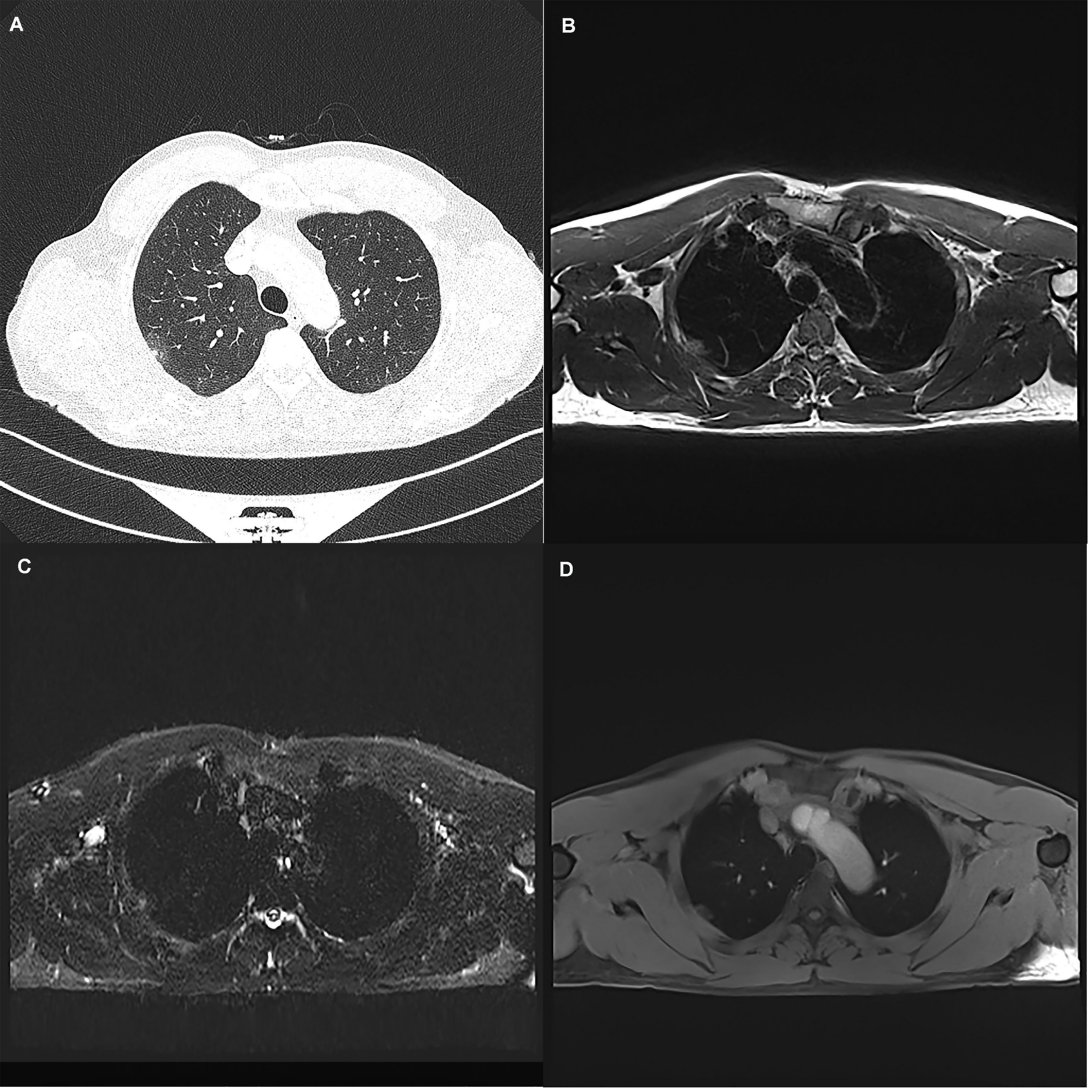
A 45-year-old woman with a 19 mm GGN (arrow) in the right upper lobe. The nodule is clearly seen on the computed tomography image (A) and the magnetic resonance image sequences T2-BLADE (B), T2-HASTE (C), and T1-Star-VIBE (D). The arrows represent the location of the nodule.
